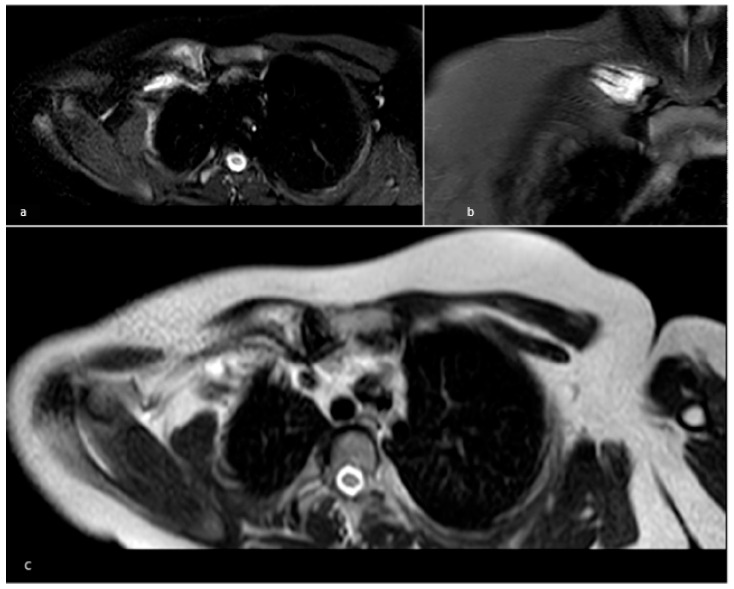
Patient 6, diagnosed with LCH. (a) Whole-body MRI showing a focal area of hyperintensity in the long TR sequences (STIR image) at the medial third of the right clavicle with cortical swelling and periosteal reaction; (b) Coronal STIR image clearly showing the hyperintensity area in STIR; (c) T2-weighted sequence highlighting both the morphological and signal alteration (hyperintensity) of the medial third of the right clavicle.
